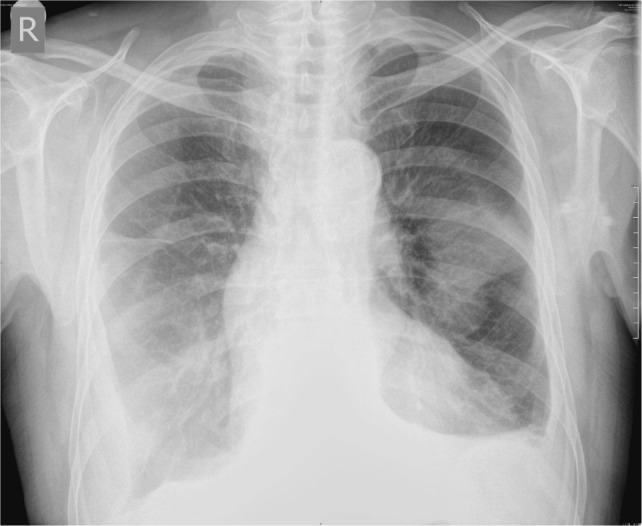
Initial chest radiography.
